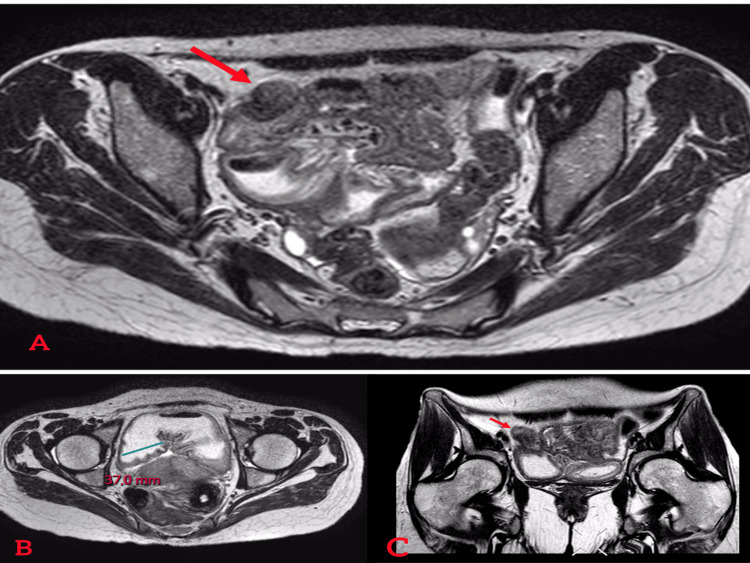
MRI findings. (A) Axial image showing an irregular and assymetric ileal stricture (arrow). (B) Terminal ileum distension. (C) Coronal image showing the ileal stricture (arrow).
